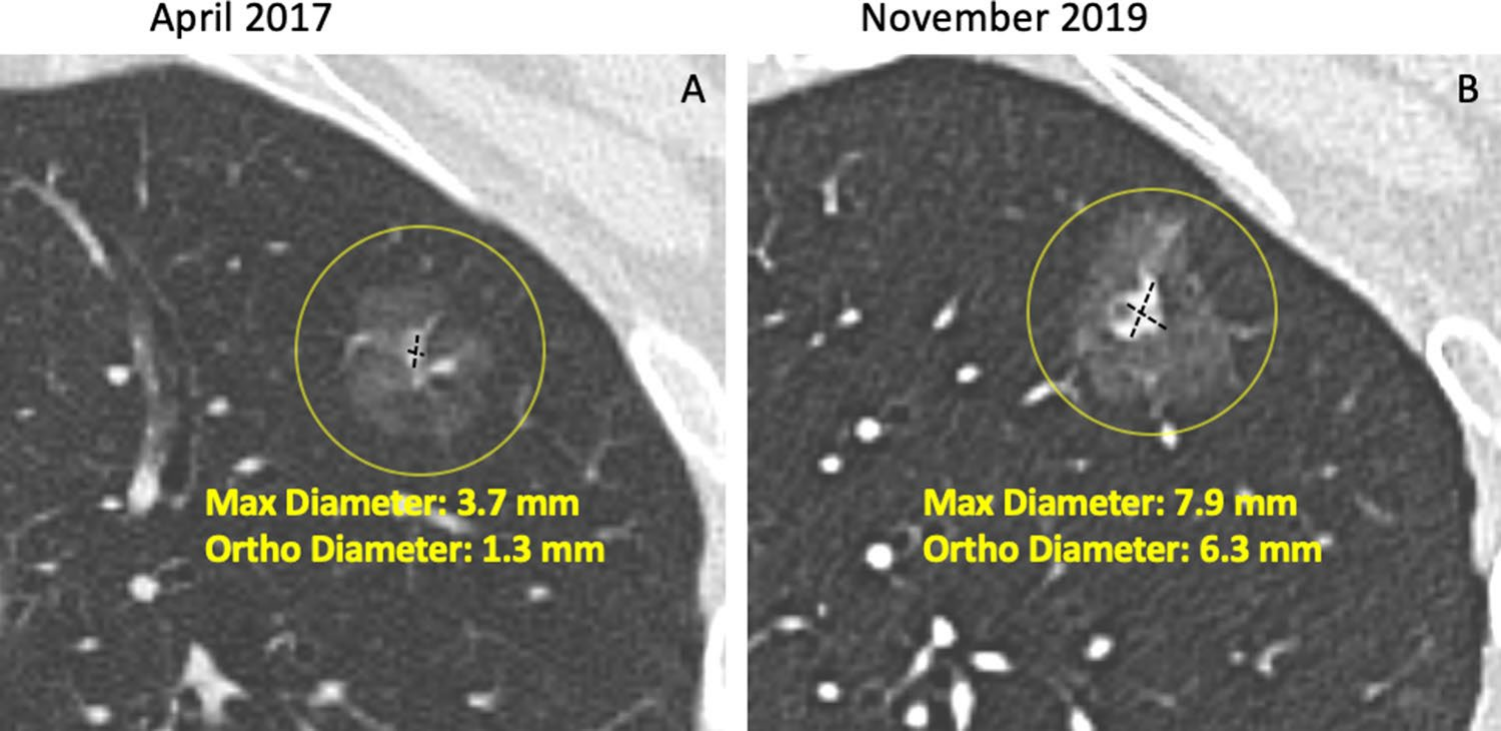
A–B. Measurement of a part-solid nodule in the left upper lobe and its growth. Axial CT image showing a part-solid nodule in the left upper lobe. The size of the solid component by manual caliper at first detection (A: maximum diameter 3.7 mm, orthogonal diameter 1.3 mm, mean diameter 2.5 mm) is thereafter confidently increased at follow up scan (B: 7.9 × 6.3 mm, mean diameter 7.1 mm). The variable and limited density difference between solid component and non-solid component represents a factor for variability of semi-automated volume segmentation. Moreover, the figure shows small vessels abutting the surface of the solid component, that is one common factor that further hampers the use of volume segmentation of solid core in part-solid nodules
